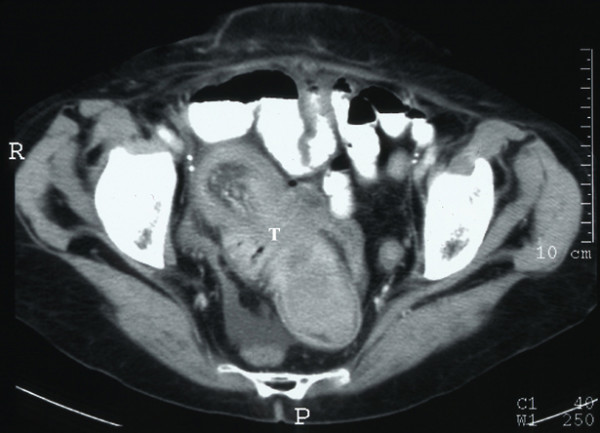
Contrast-enhanced computed tomography scan demonstrating mild dilatation of the ileum proximal to the level of the intussusception with a 'telescoping' appearance of the small bowel intussusception distal to this area (T).
